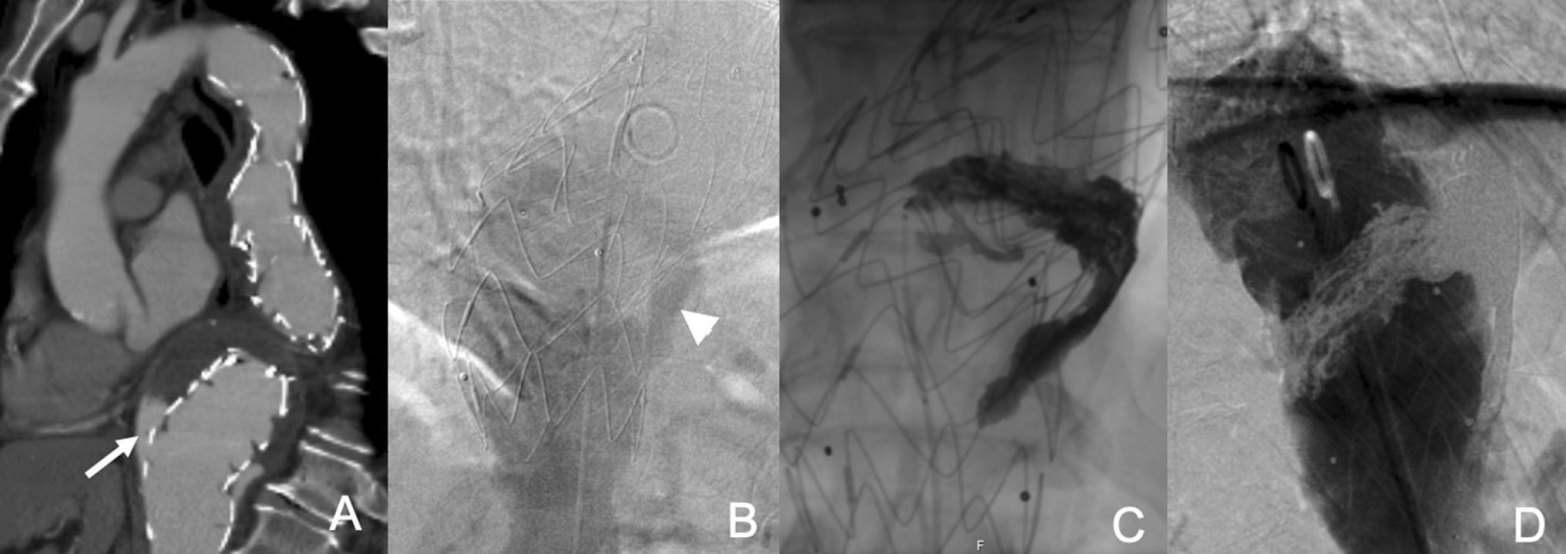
EL1b embolisation following TEVAR. A Sagittal CT angiogram shows distal EL1 in patient with a thoracic endograft (arrow). B Aortic angiograms confirm EL1b (arrow head). C Embolisation of the endoleak cavity with via a microcatheter. D Final angiogram shows successful endoleak embolisation
